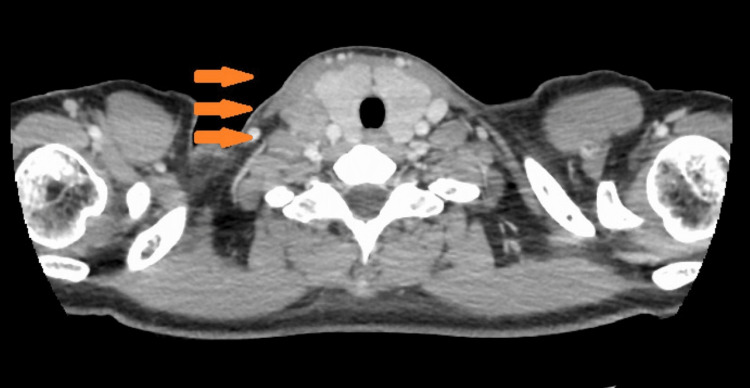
CT of the neck. Supraclavicular fossa lymph node at the initial examination.
